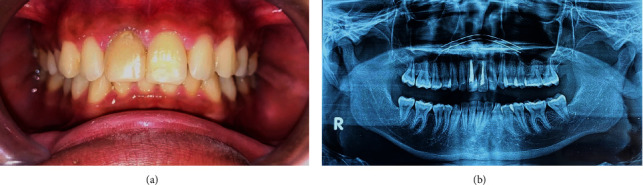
6 months postoperative recall: (a) intraoral view; (b) radiographic examination.
